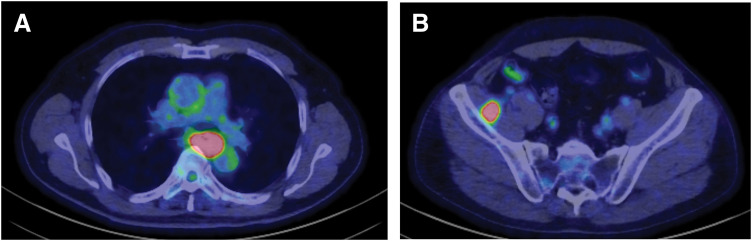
PET-CT at initial diagnosis. (A) PET-CT demonstrates FDG uptake in the primary esophageal lesion. (B) PET-CT shows intense FDG uptake in the iliopsoas muscle consistent with distant metastasis. FDG, fluorodeoxyglucose
